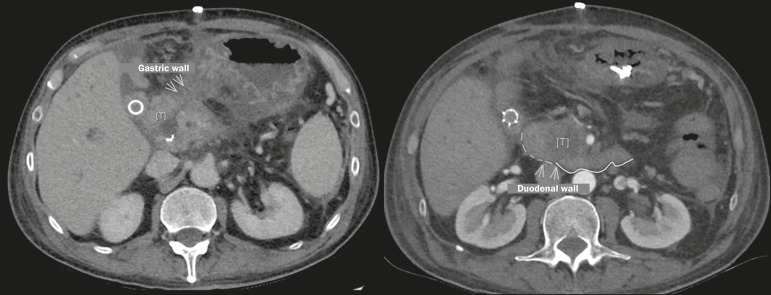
Contrast-enhanced abdominal CT scans, in the axial plane, showing a
